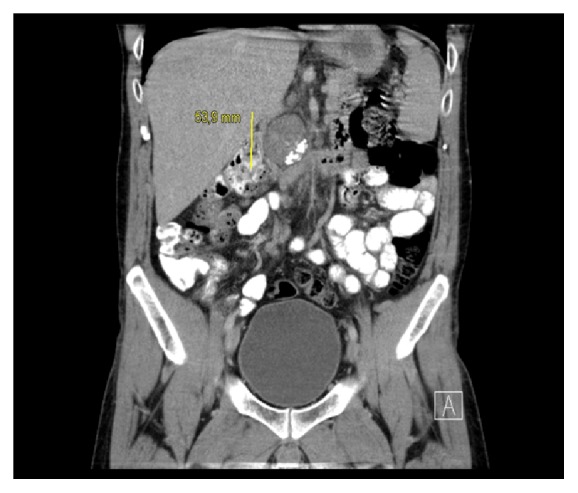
Abdominal CT scan showing a 5 × 6 cm mass with several calcifications between abdominal aorta and vena cava below the left renal vein: location is identical with metastatic site at first presentation.
